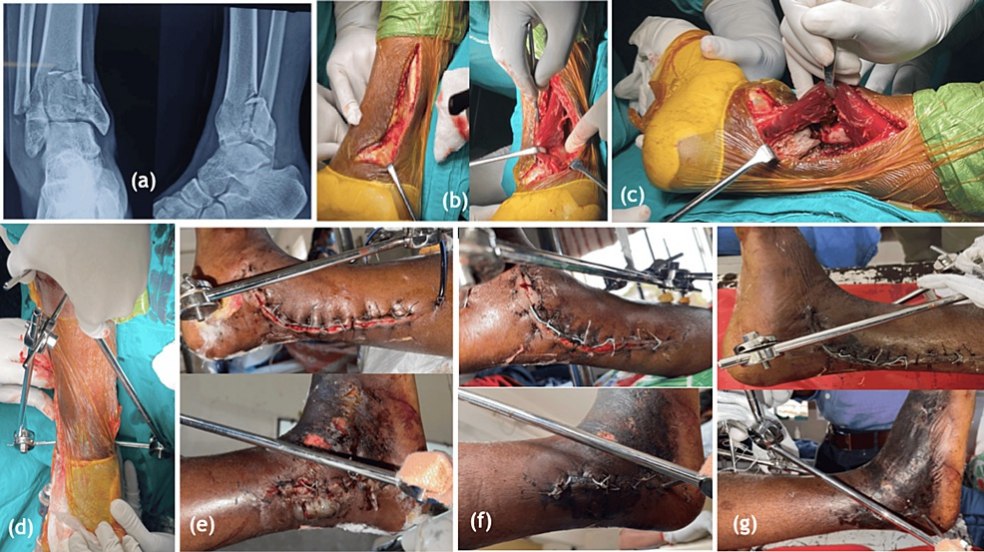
Complication of delayed wound healing and development of blebs. (a) AP and lateral radiographs on presentation, (b) posteromedial approach, (c) elevation of FHL muscle to reduce fracture and plate fixation, (d) external fixation for additional stability, (e) development of blisters and swelling on POD 2, (f) Skin condition on POD 7, no blisters, (g) Skin condition on POD 16 AP: antero posterior; POD: postoperative day
